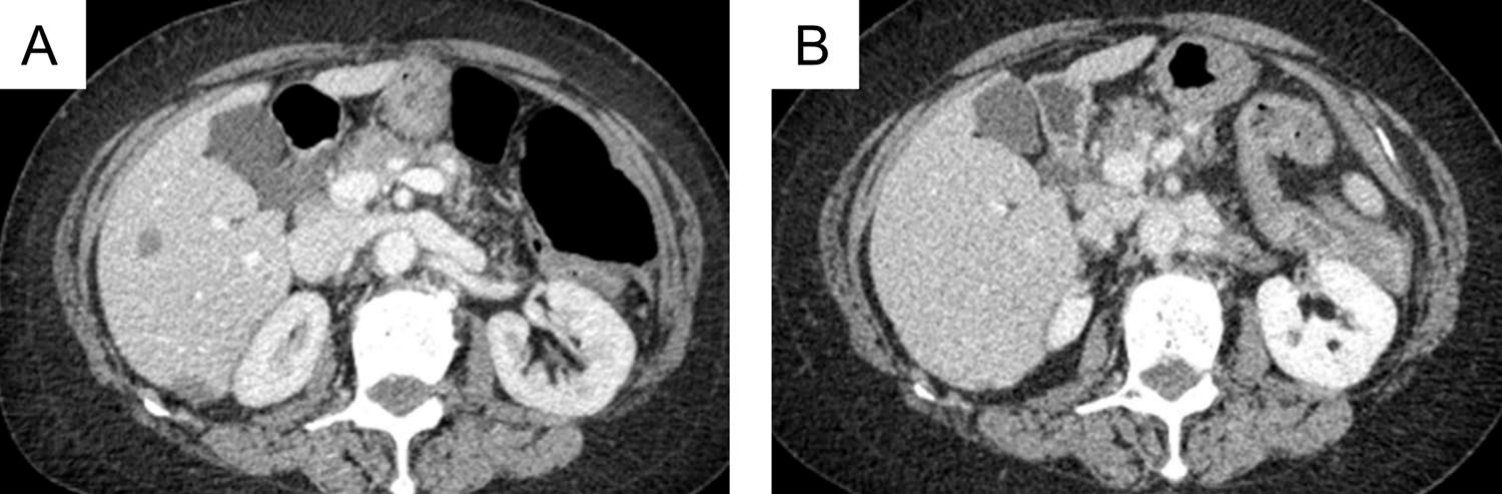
Imaging of the abdomen after methotrexate withdrawal. CT of the abdomen four months later revealed spontaneous shrinkage of most of the tumors (A). There has been no sign of recurrence for eight months (B)
